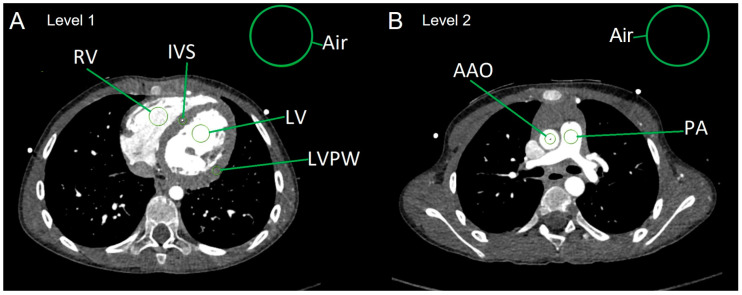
Measurement of CT attenuation values with regions-of-interest (A) at level 1 (IVS: interventricular septum; LV: left ventricle; LVPW: LV posterior wall; RV: right ventricle) and (B) at level 2 (AAO: ascending aorta; PA: main pulmonary artery). Standard deviation of the attenuation values obtained from the ROI placed in the air outside of the body served as background noise.
